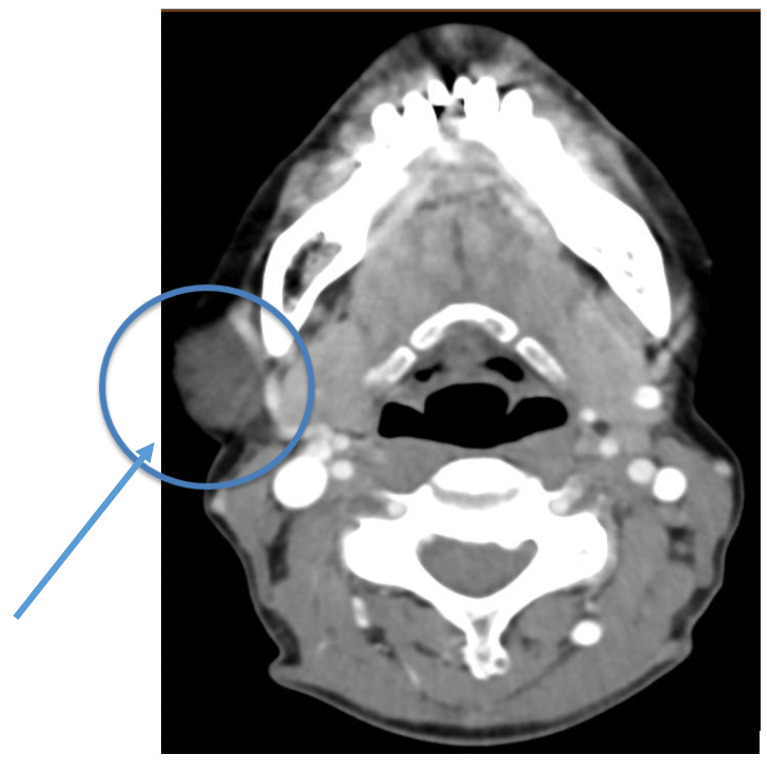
Contrast-enhanced axial section of pre-operative cervical Computed Tomography (CT) scan, demonstrating a well circumscribed middle attenuation mass with peripheral blood vessels enhancement, located in proximity to the superficial parotid lobe (round blue line and arrowhead).
